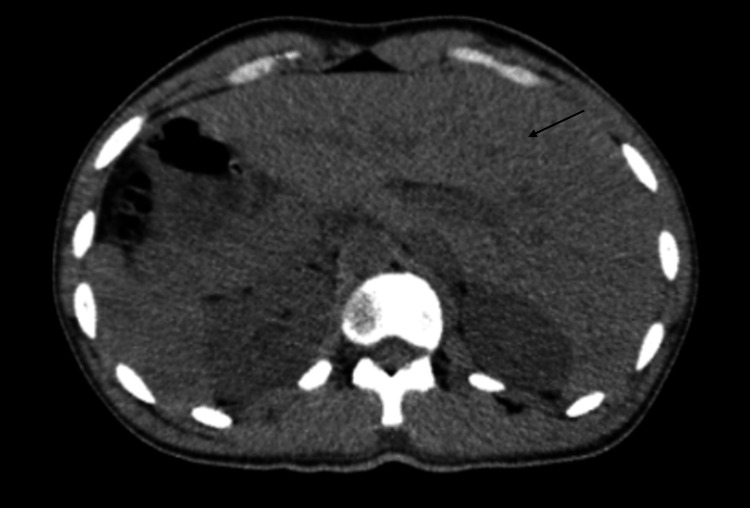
CT abdomen and pelvis w/o contrast (axial view): situs inversus and dextrocardia. No acute abnormality in the abdomen or pelvis; bronchitis and bronchiolitis; left-sided liver (situs inversus), and the gallbladder is not distended.
